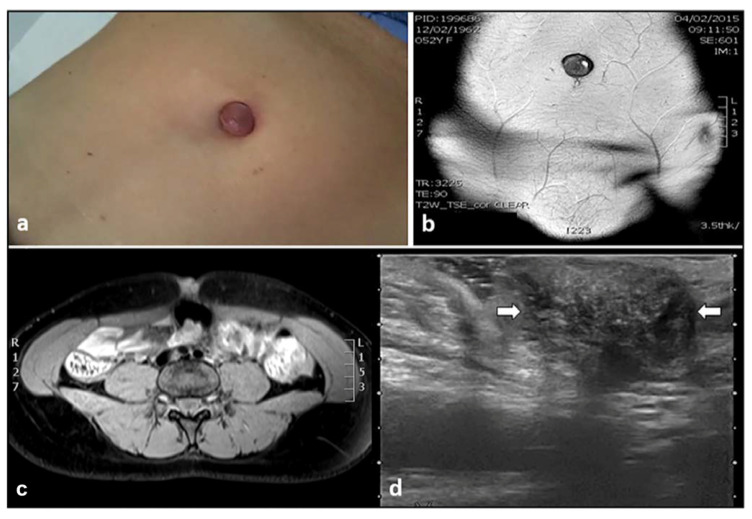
Umbilical mass, identified during physical examination (a), studied with MRI (b,c), and ultrasound imaging (d). White arrows in d revealed a hypoechoic hernial sac and intralesional hyperechoic spots, representing an umbilical hernia with endometriosis foci.
